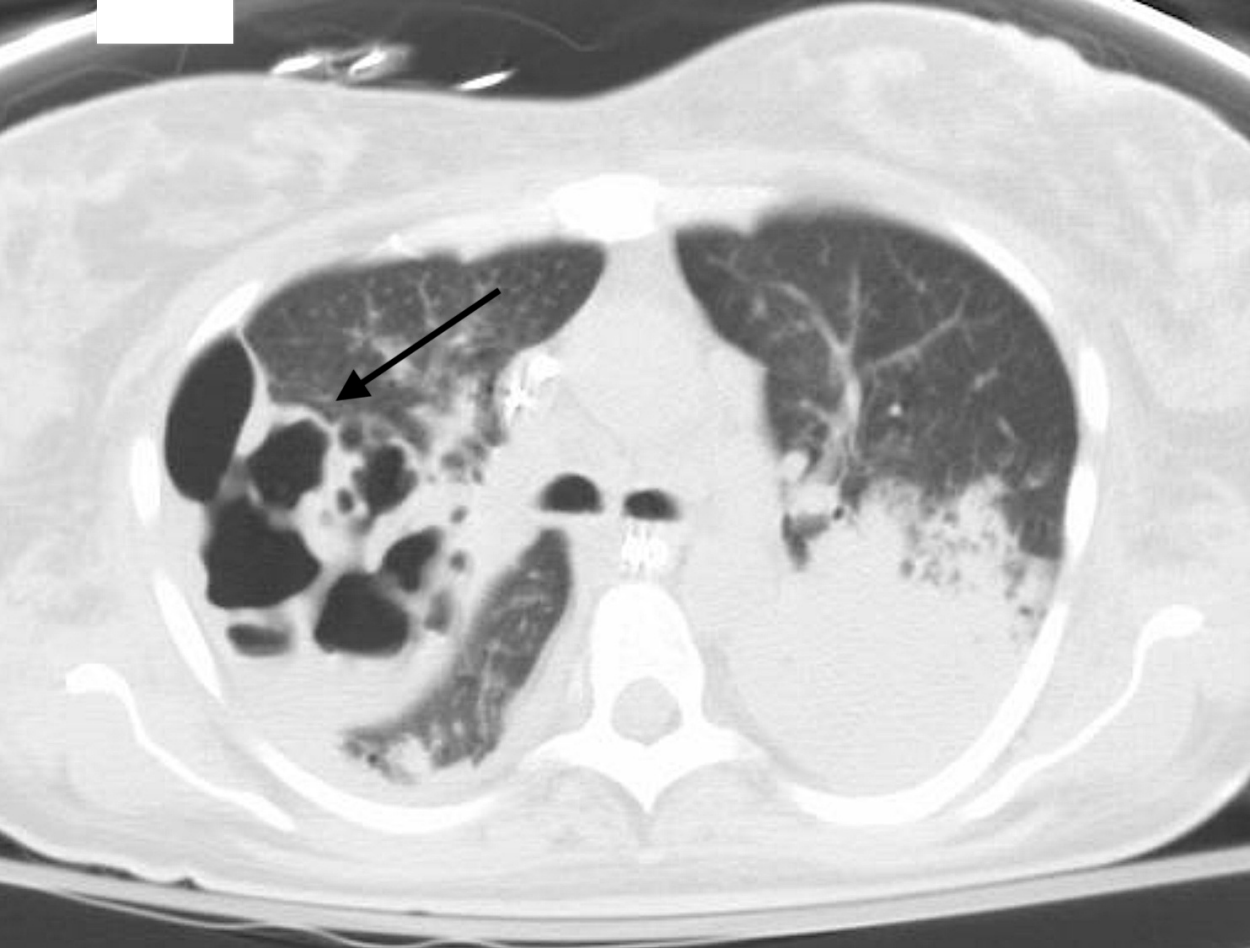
Computed tomography (CT) chest (axial view) showing worsening and multiple cavitary lesions in the right lung (arrow).
